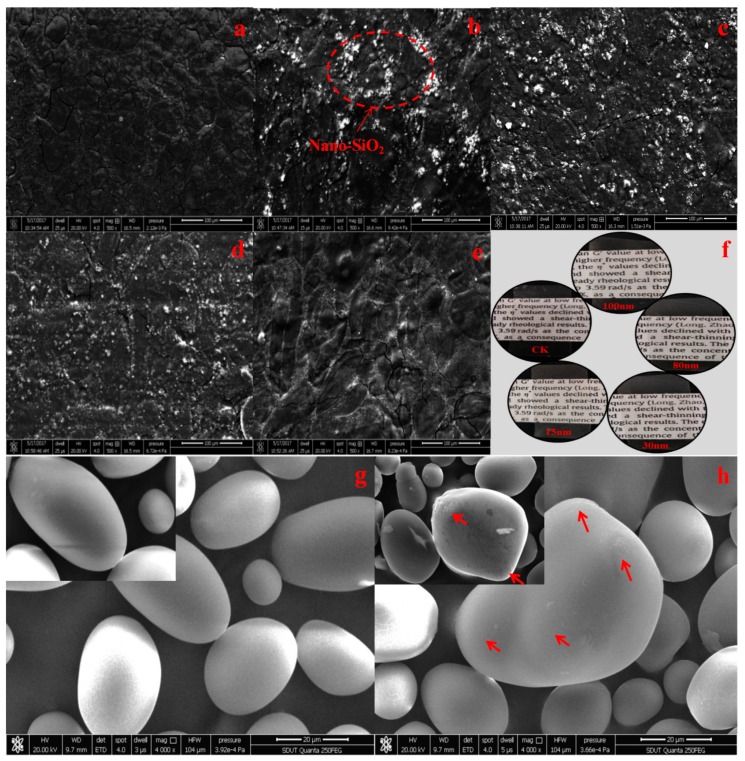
SEM and apparent images of starch and nano-SiO_2_/potato starch films. (a) CK: films without nano-SiO_2_; (b) films with the 15 nm nano-SiO_2_; (c) films with the 30 nm nano-SiO_2_; (d) films with the 80 nm nano-SiO_2_; (e) films with the 100 nm nano-SiO_2_; (f) the apparent images of different films (g) native potato starch; (h) potato starch after ultrasonication.
